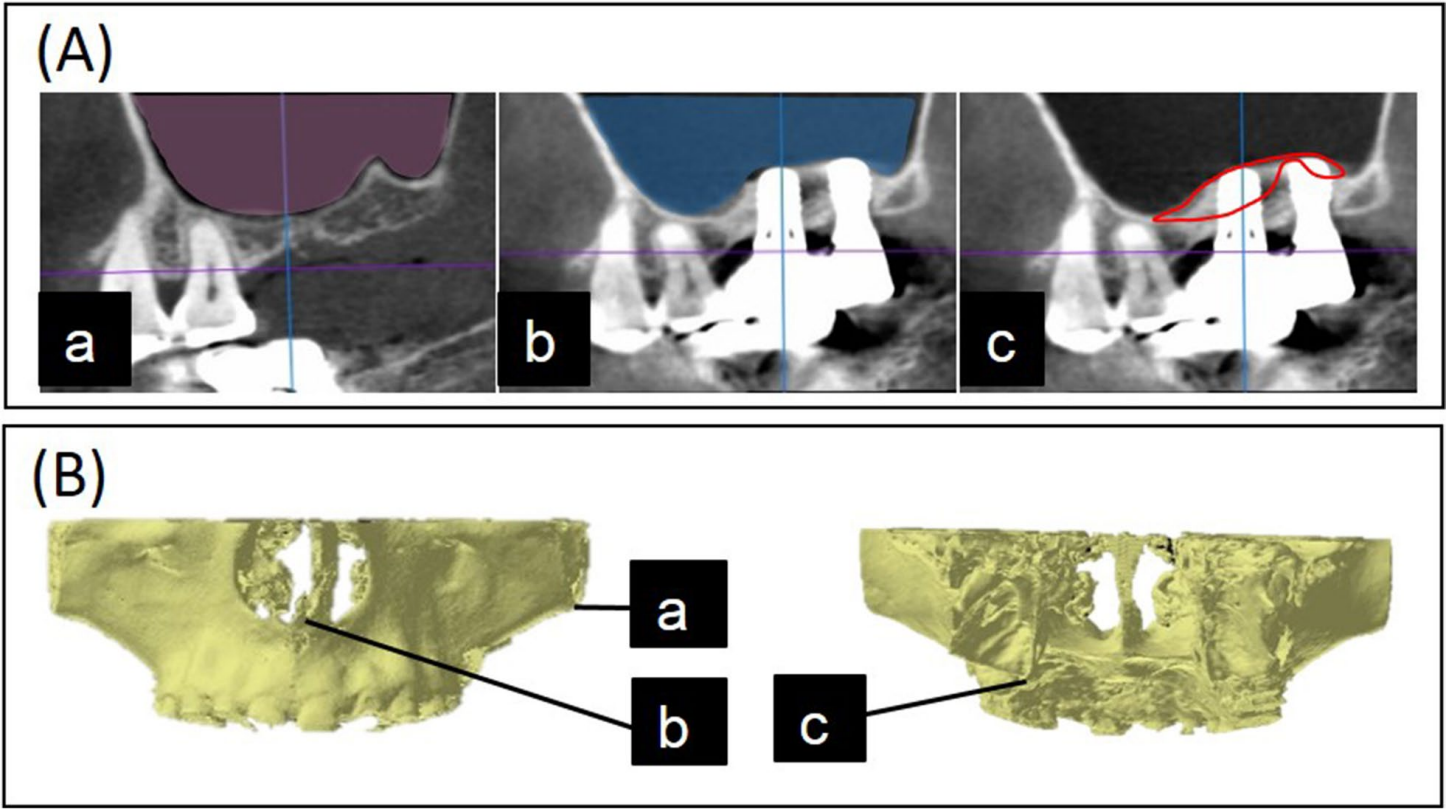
A Cone-beam computed tomography (CBCT) analysis. a An image of the maxillary sinus was extracted from the Digital Imaging and Communications in Medicine (DICOM) files of CBCT at T0. b An image of the maxillary sinus was extracted from the DICOM files of CBCT at T1, T2, or T3. c After deleting the overlapping part from before and after surgery, the remaining part (in the red line) was measured. B Reference points for superimposition. a The zygomatic process of maxilla. b The anterior nasal spine of maxilla. c The pterygoid hamulus of sphenoid bone
